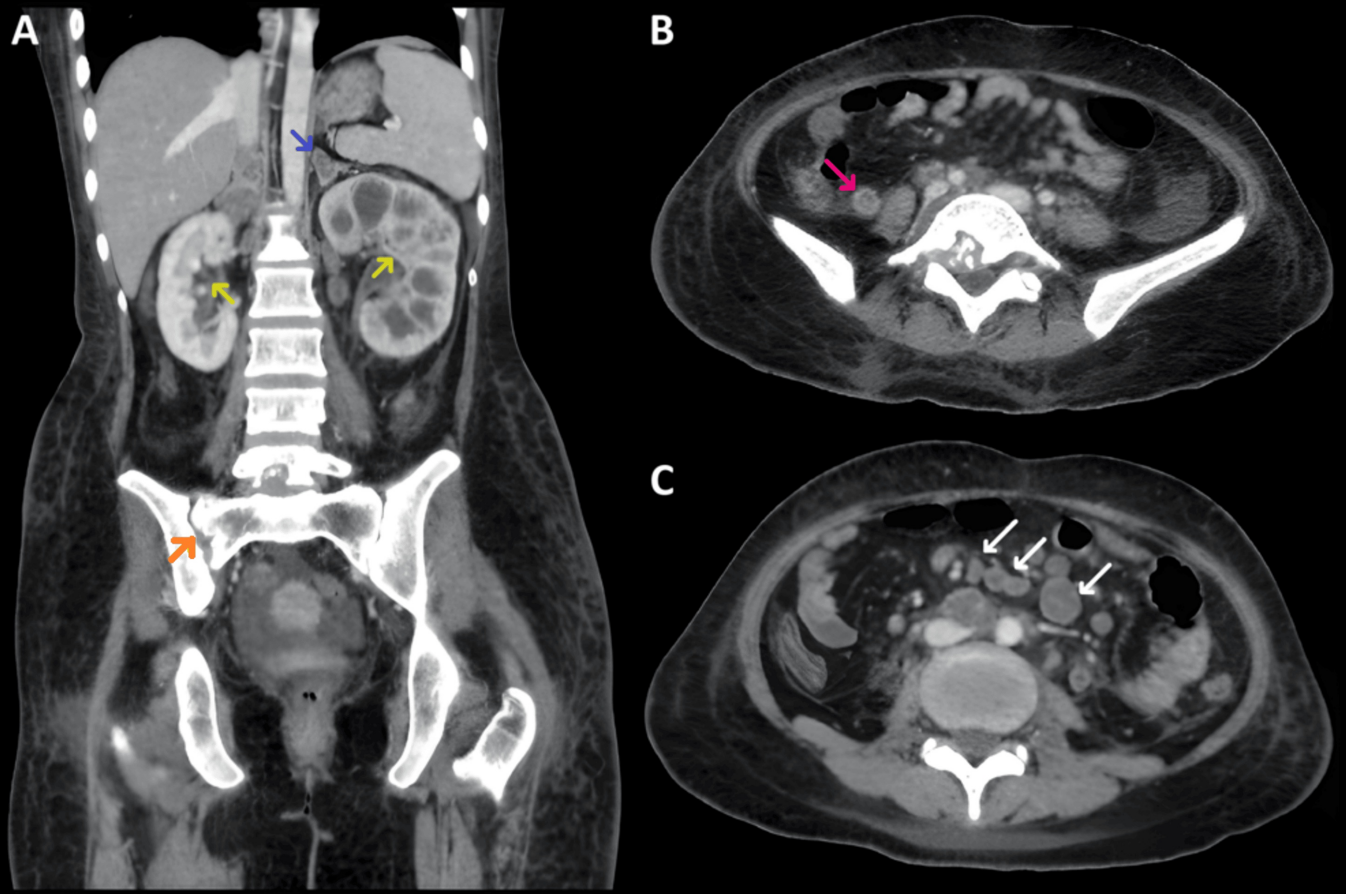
CECT of the abdomen Pane (A): Bilateral bulky adrenal glands with large conglomerate, peripherally enhancing, hypodense lesions within (blue arrow); bulky left kidney with moderate hydronephrosis (green arrows); and infective right sacroiliitis with L5 lytic lesion (orange arrow). Pane (B): Mild thickening of the ileocecal junction and cecum, with a secondarily inflamed appendix (pink arrow). Pane (C): Necrotic abdominal lymphadenopathy (white arrows). CECT, contrast-enhanced computed tomography
